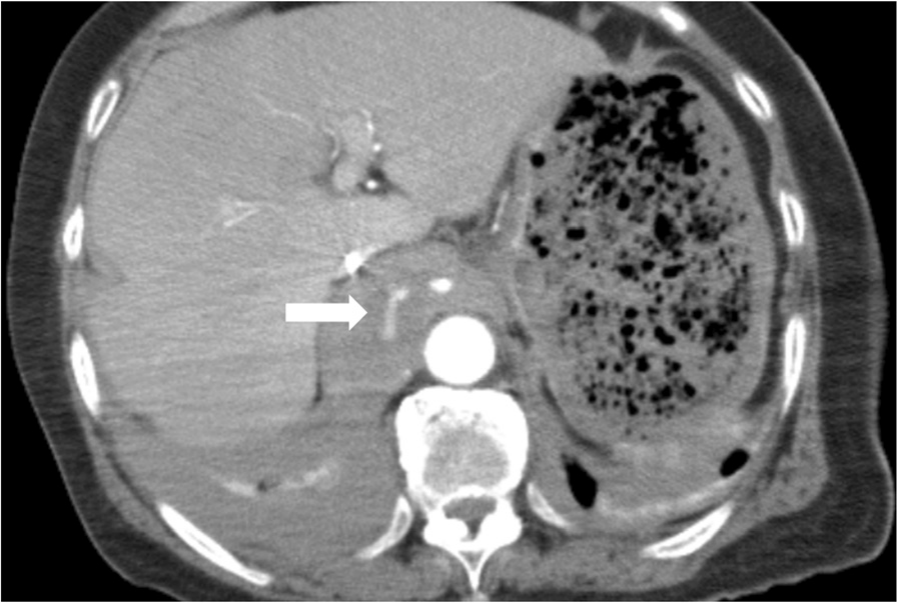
Enhanced CT showed the extension of the hematoma from within the retrocrural space to the right pleural space, and the extravasation of the retrocrural space (arrow)
